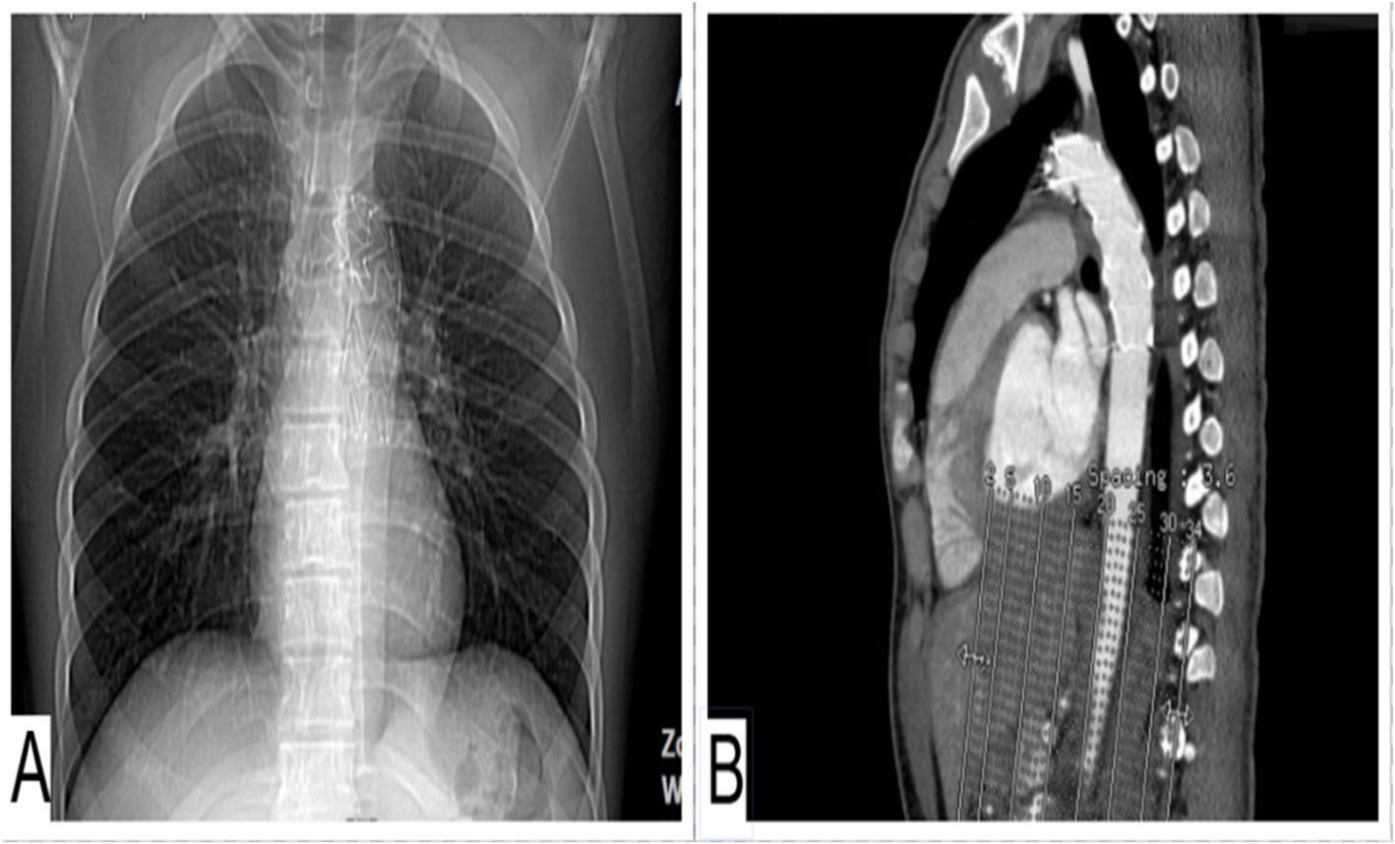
Follow-up Chest X-ray (A) and CT angiography (B) 45 days after the implantation of the stent and debranching surgery
